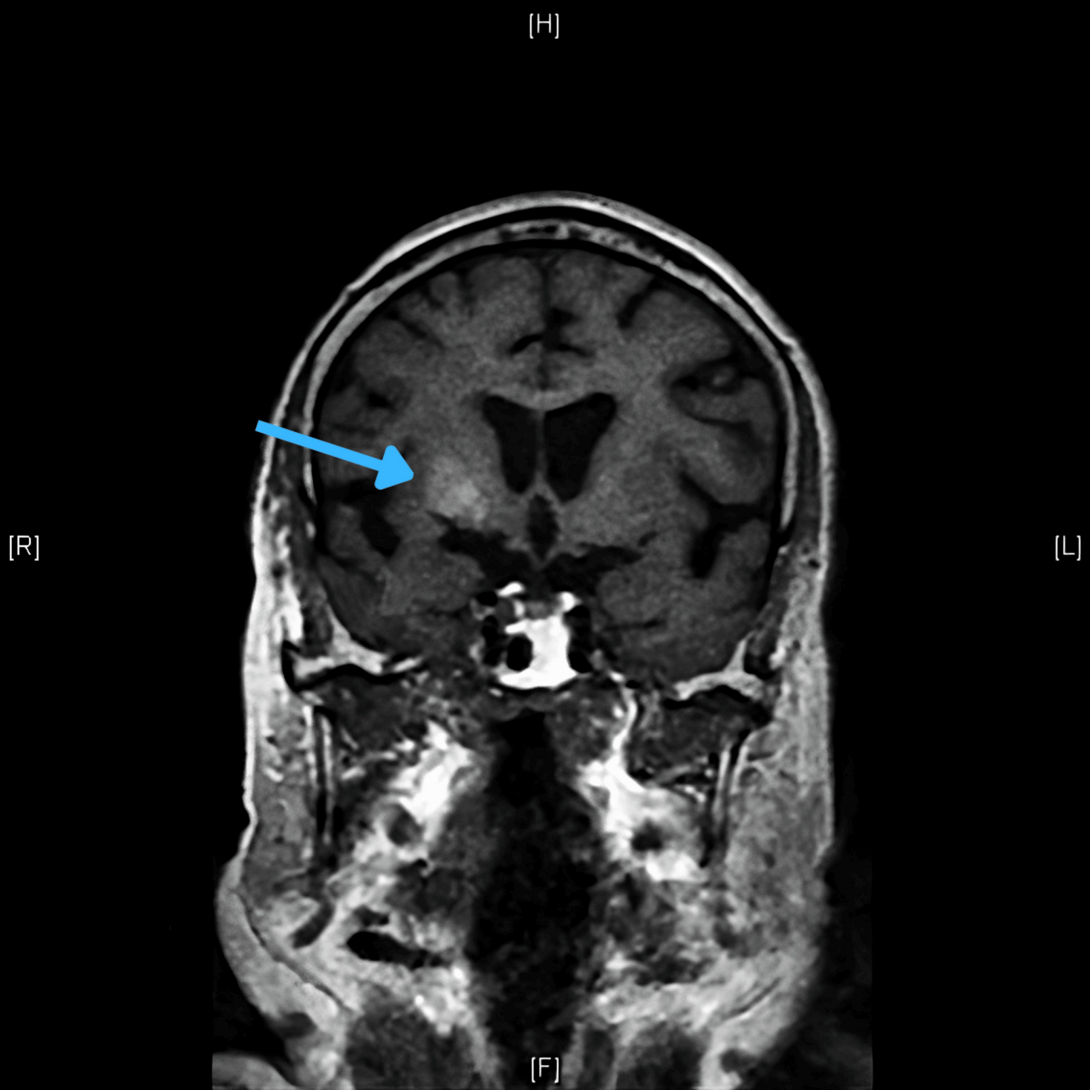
MRI with frontal view of T1 weighting highlighting spontaneous hypersignal in the affected basal ganglia (contralateral to the choreiform limb).
